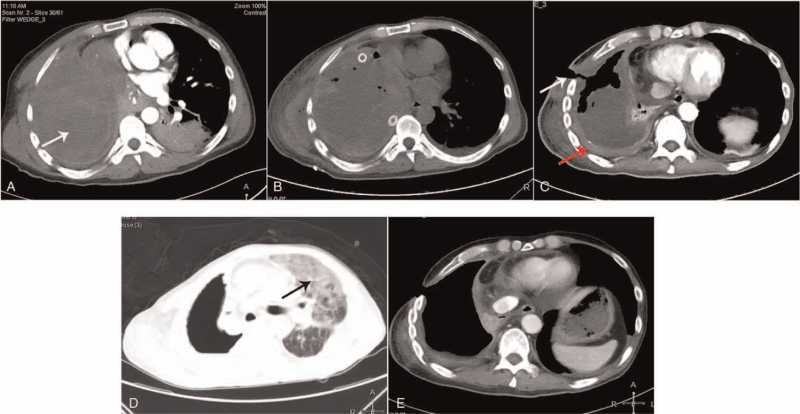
(A) CT images demonstrate a large mass (arrows) in the right thoracic cavity before emergency surgery (arrows). (B) CT images show pleural effusion and atelectasis of the right lung on the 12th postoperative day. (C) CT images show the chest sinus tract (white arrows) and thickening of pleural lesions (red arrows) after completing 2 cycles of nab-paclitaxel treatment. (D) CT images demonstrate grade 3 immune-associated pneumonia (arrows) after completion of the first cycle of pembrolizumab. (E) CT images demonstrate stable disease after completion of 7 cycles of pembrolizumab.
